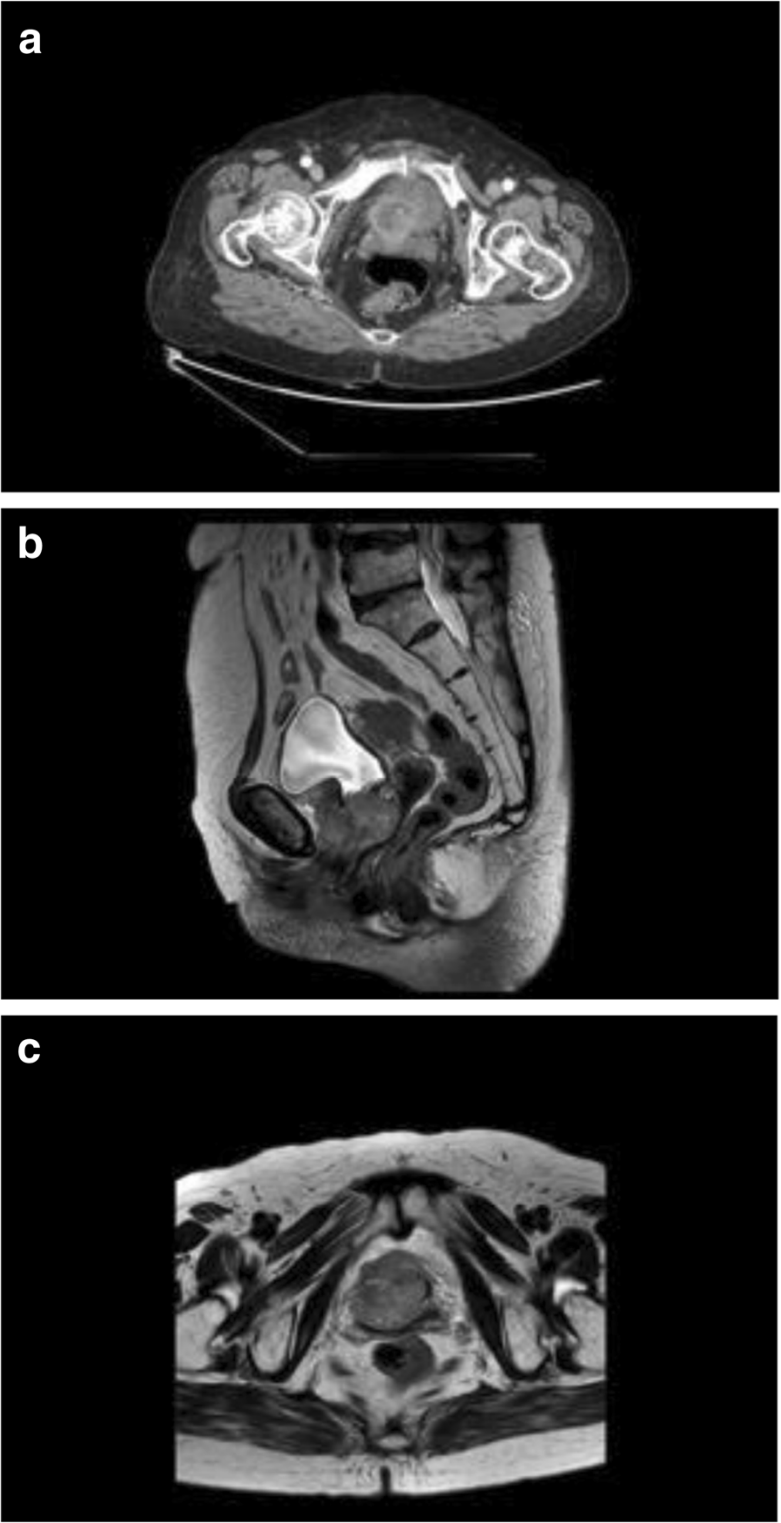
Preoperative gynecologic MRI and abdominal CT images. a A 4-cm recurred tumor involving the bladder neck, urethra, and upper vagina (horizontal view, abdominal pelvis CT). b An increased recurred tumor involving the bladder neck, urethra, and upper vagina (4.6 cm). There were no newly appearing lesions in the pelvic cavity indicating a lack of metastasis (sagittal view, MRI gynecology). c. A 4-cm recurred tumor involving the bladder neck, urethra, and upper vagina. There were no other newly appearing lesions in abdominopelvic cavity indicating a lack of metastasis (horizontal view, MRI gynecology)
